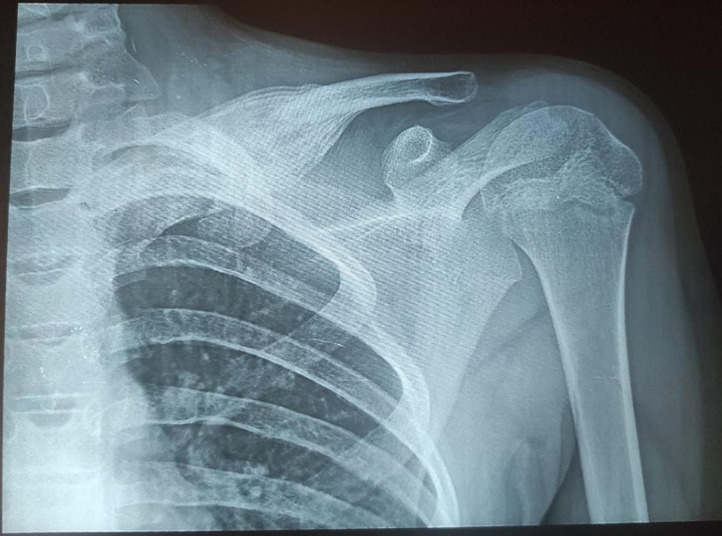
anteroposterior control shoulder X-ray: periosteal clavicle apposition with “onion bulb” shape lesion
